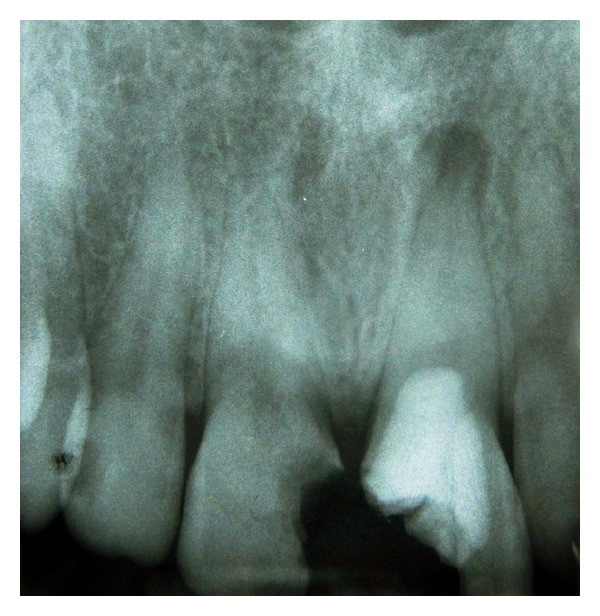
Preoperative radiograph; root canal of the left maxillary central incisor has been over-prepared and periapical radiolucency is observed at the apex of the tooth.
